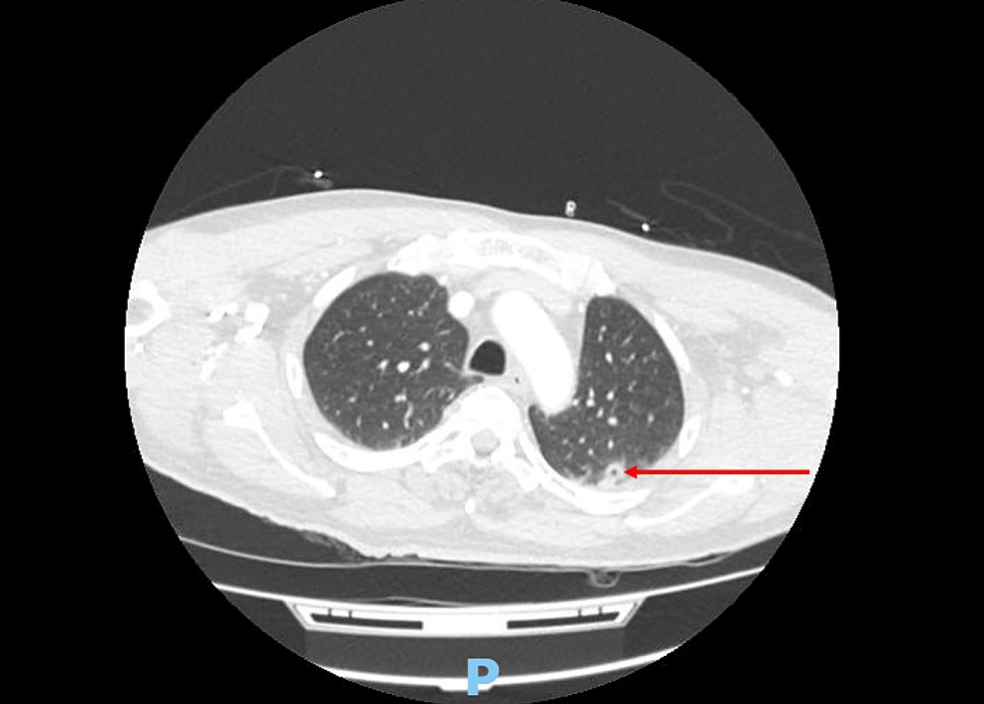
Chest computed tomography showing a cavitating nodule in the left lung apex.
